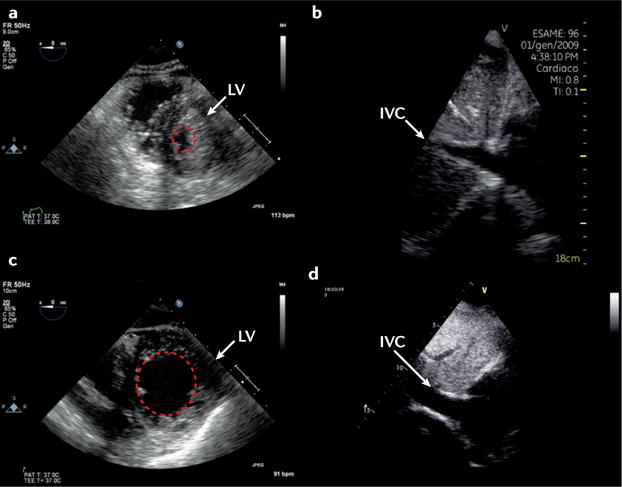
Static 2D echocardiography parameters are used to evaluate potential volume responsiveness The upper panels show a patient who is severely hypovolaemic, and responded to volume loading with an increase in stroke volume. a | Short-axis view of the left ventricle (LV) is shown, where the left ventricular end-diastolic area (dotted red circle) is small. b | From a subcostal view, an obliterated inferior vena cava (IVC) at end-expiration (<1 cm) can be observed. The lower panels show a patient who, according to static 2D echocardiography parameters, would not be predicted to respond to volume loading by increasing stroke volume. c | Short-axis view of the LV with a normal left ventricular end-diastolic area (dotted red circle). d | Dilated IVC at end-expiration.
